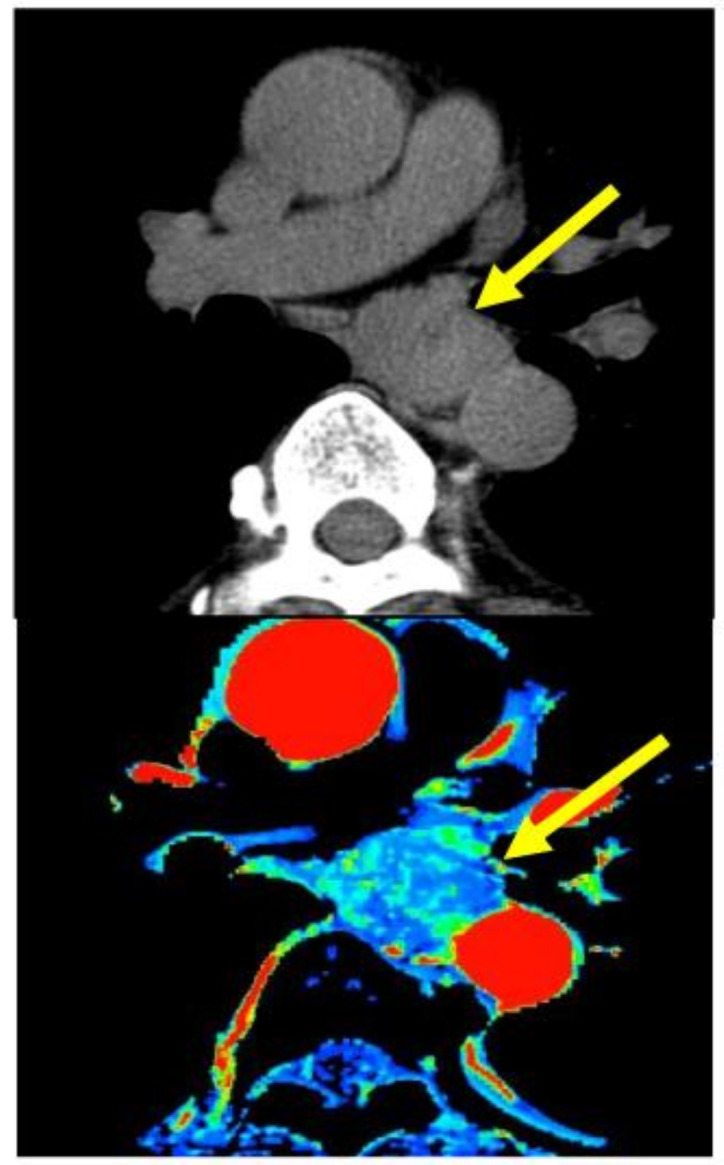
(Upper) plain CT image. (Lower) Calculated image using perfusion-CT for esophageal squamous cell carcinoma. Arrows indicate the esophageal tumor.
